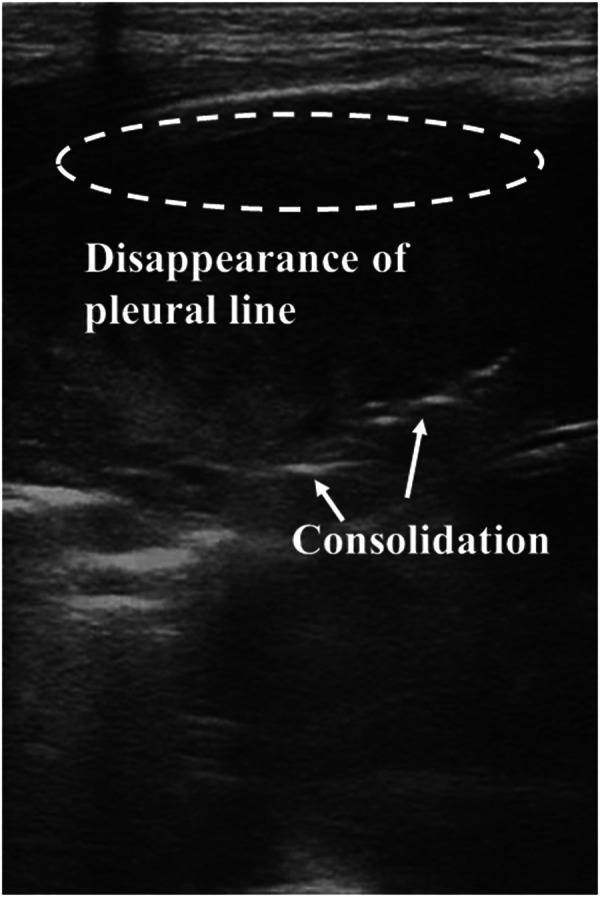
Pneumonia on lung ultrasonography. Ultrasound shows irregular areas of lung consolidation with air bronchograms, disappearance of the pleural line, and A-lines.
